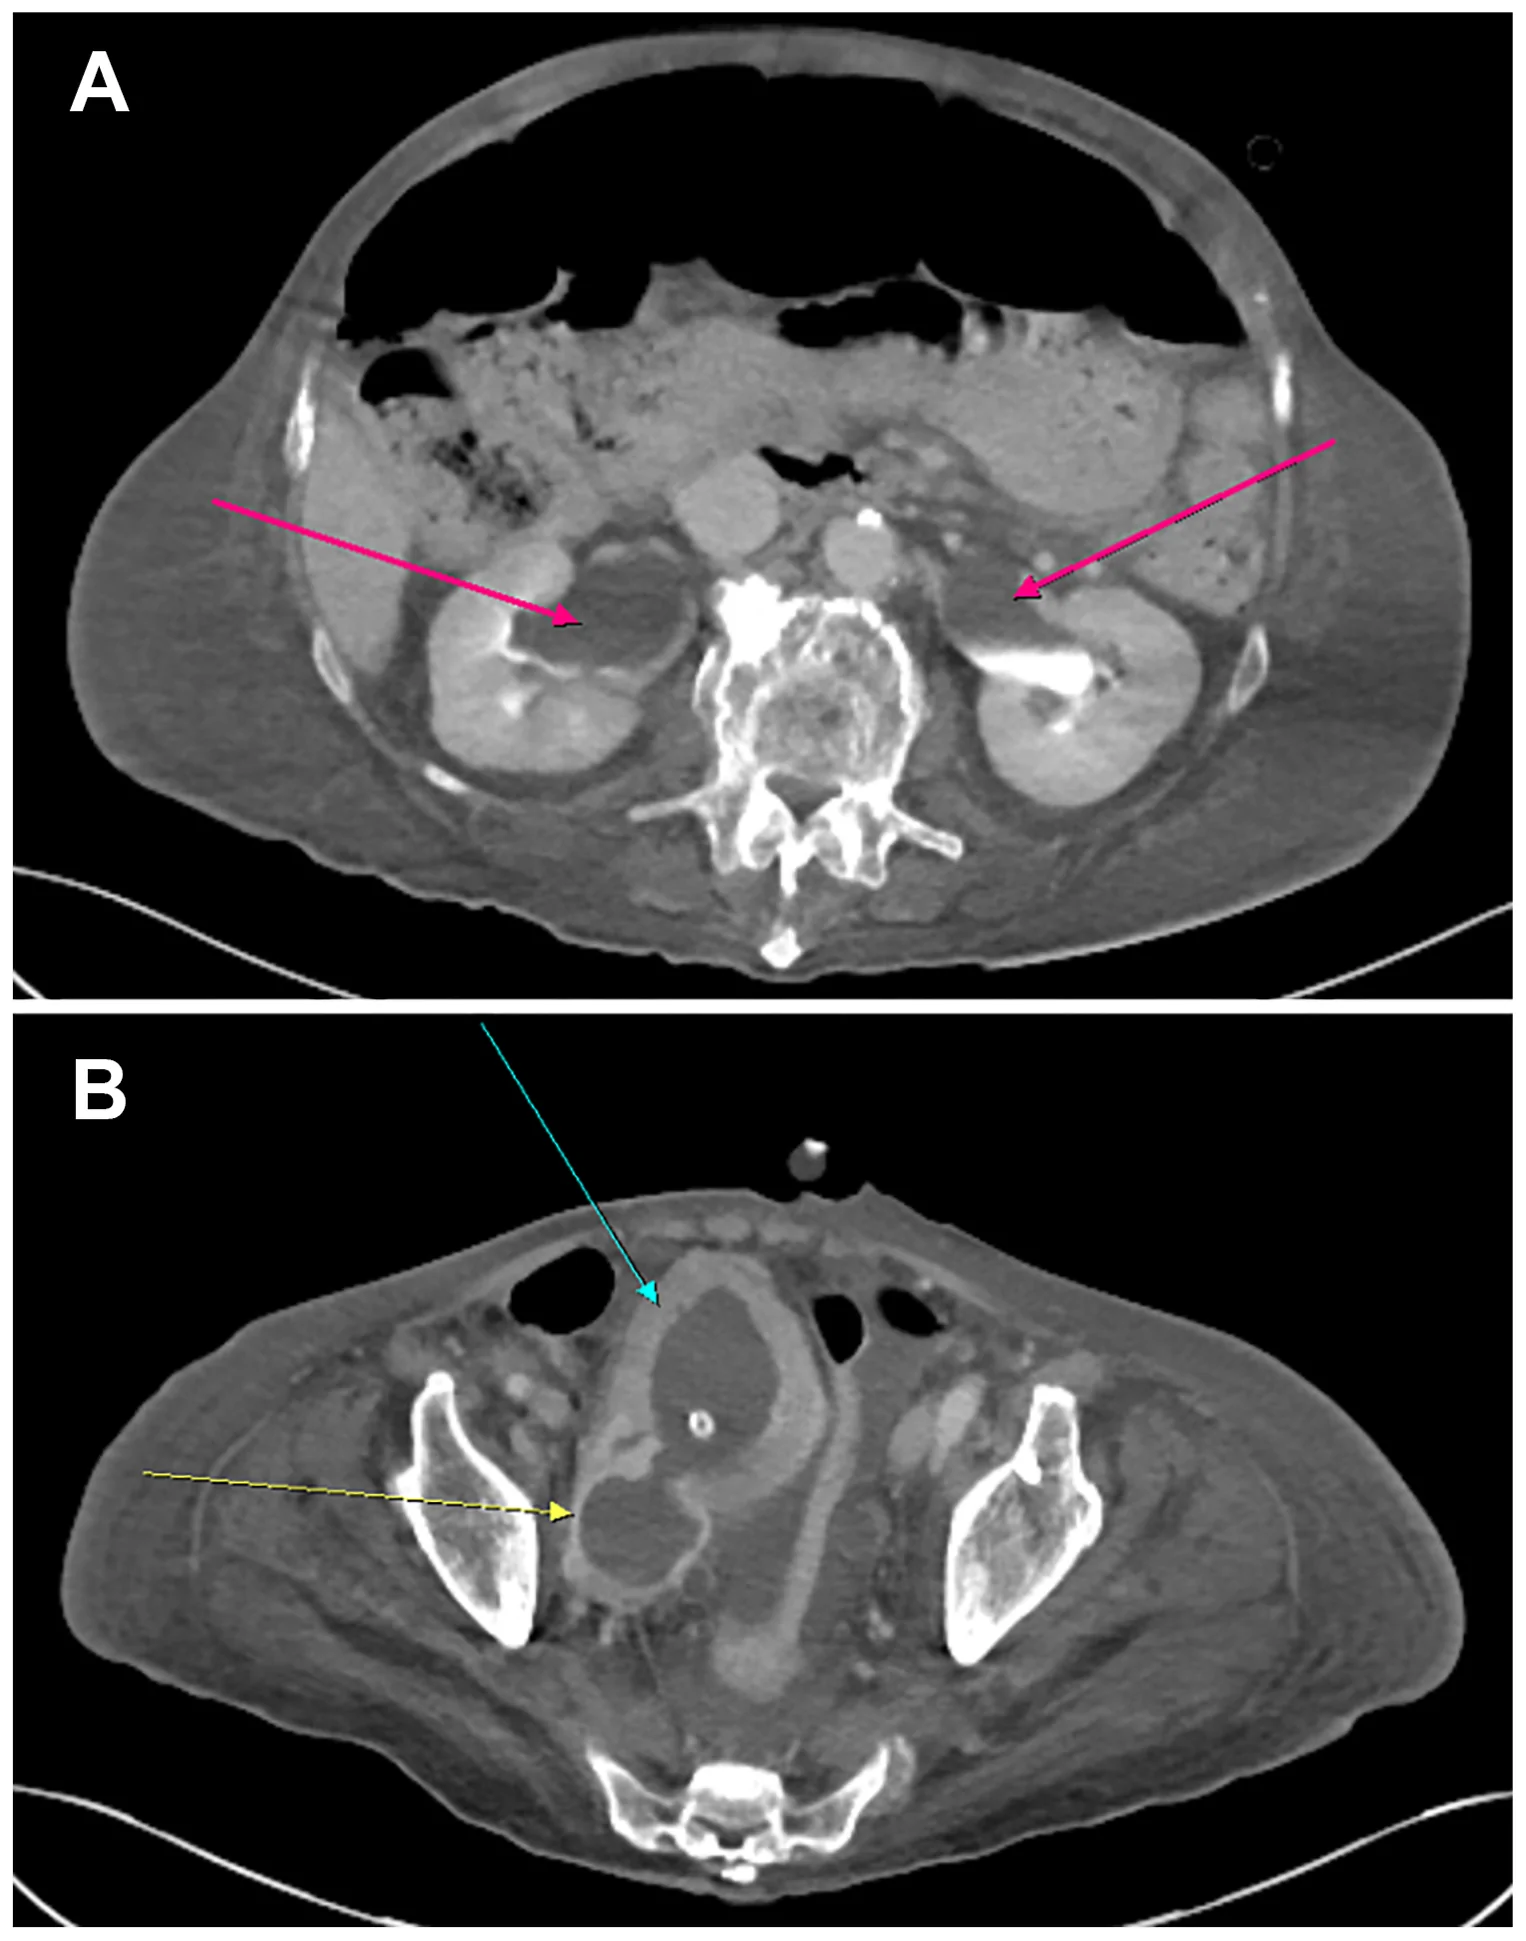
Computed tomography findings suggestive of obstructive uropathy and urinary tract infection. A, Venous phase axial computed tomography image showing bilateral hydronephrosis with retained contrast material in the dilated renal collecting systems (*magenta arrows*). B, Axial computed tomography image showing bladder wall thickening suggestive of cystitis (*light blue arrow*) and a bladder diverticulum (*yellow arrow*).
